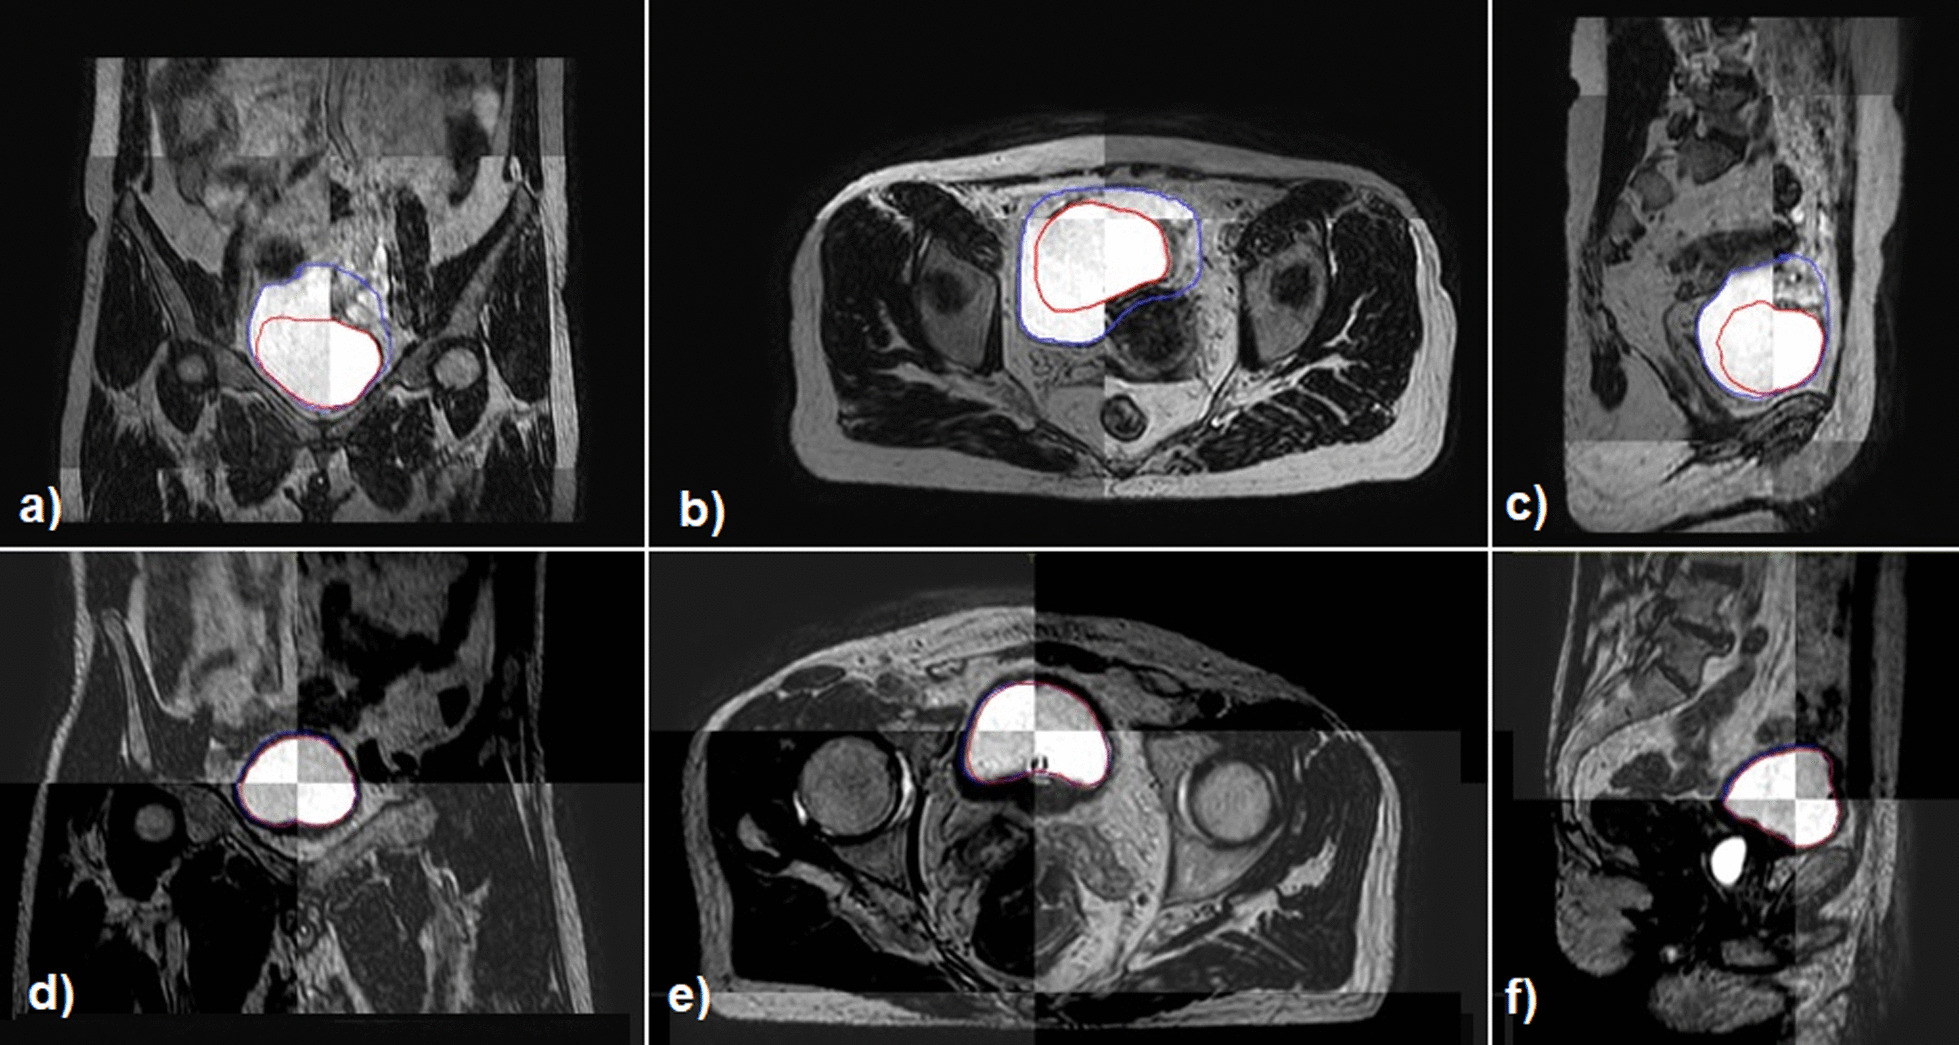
Bladders contours on MRI scans acquired at the beginning and the end of a 1.5 T MR-Linac online adaptive session and rigidly registered. Coronal, transversal and sagittal MRI planes for a representative patient not undergoing (a–c) and undergoing (d–f) the bladder filling control procedure. In red and blue the bladder contoured on the MRI acquired at the beginning and end of the session
